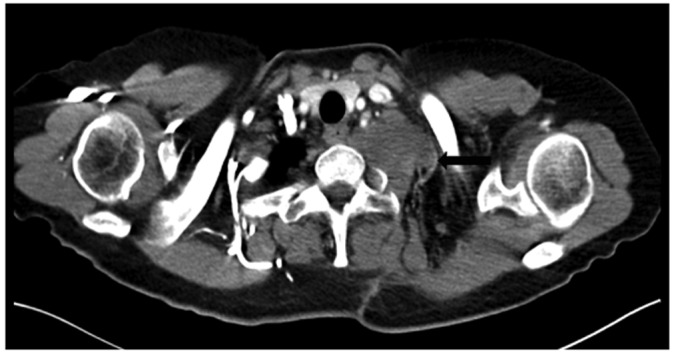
Computed tomography scan of the recurrent desmoid tumour (black arrow) wrapping around the left subclavian artery and causing erosion of the adjoining ribs in November 2012.
